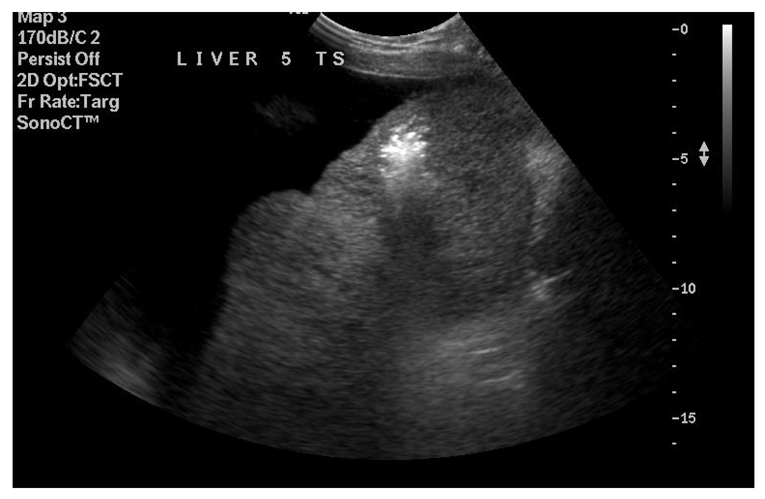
On immediate completion of ablation. With time this lesion will appear less echogenic and shrink in size minimally. Follow up imaging with a triphasic CT or MRI is needed to ensure no tumour was left behind.
